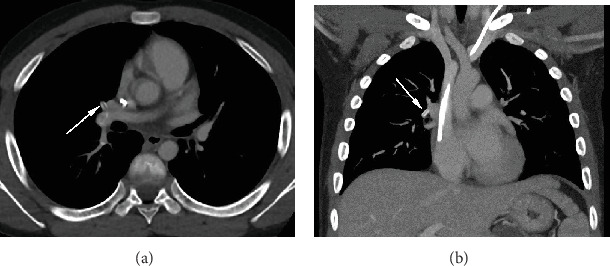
Follow-up chest CT approximately 10 months after the initial discovery of the calcified CRS demonstrates its migration to the distal right pulmonary artery (arrows) on axial (a) and coronal (b) views.
